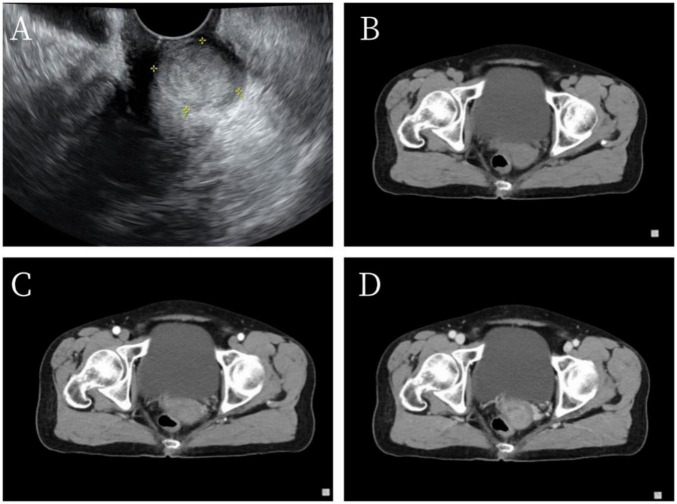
Imaging findings. (A) Vaginal ultrasonography indicated a 24 × 19-mm hypoechoic nodule in the posterior lip of the cervix with clear boundaries and heterogeneous internal echoes. (B–D) Pelvic computed tomography revealed thickening of the cervical region with persistent enhancement on early- and late-phase scans after contrast administration.
